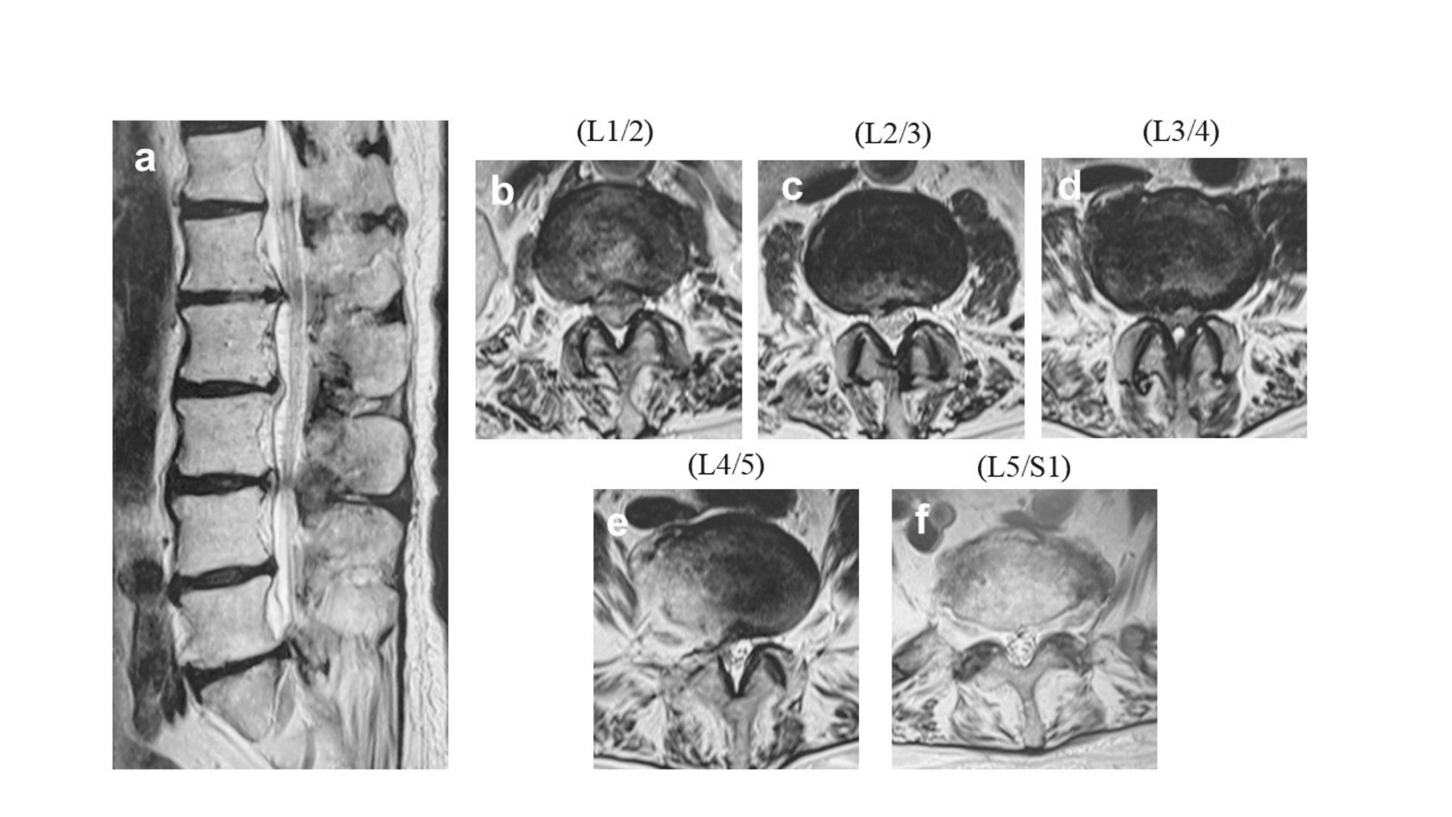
Preoperative MRI (a) Sagittal image of lumbar spine. (b) Axial image of L1/2. Lumbar spinal canal stenosis was shown. (c) Axial image of L2/3. (d) Axial image of L3/4. Lumbar spinal canal stenosis was shown. (e) Axial image of L4/5. Lumbar spinal canal stenosis was shown on the right side of L4/5. (f) Axial image of L5/S1.
